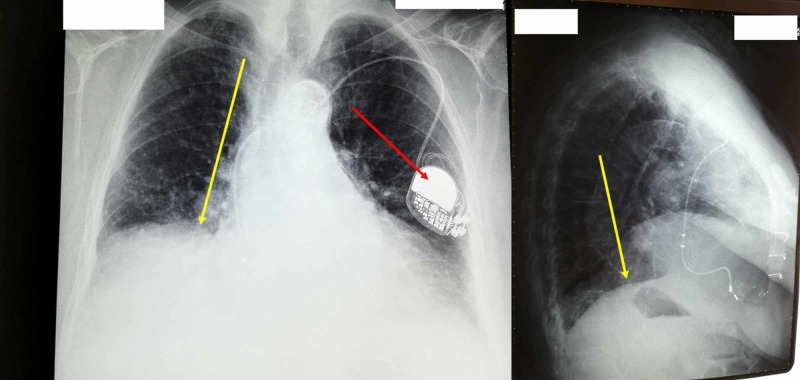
X-ray: instrumental examination showing an elevation of the diaphragm on the right side The red arrow indicates the pacemaker, the yellow arrow indicates the elevation of the right diaphragmatic dome.
